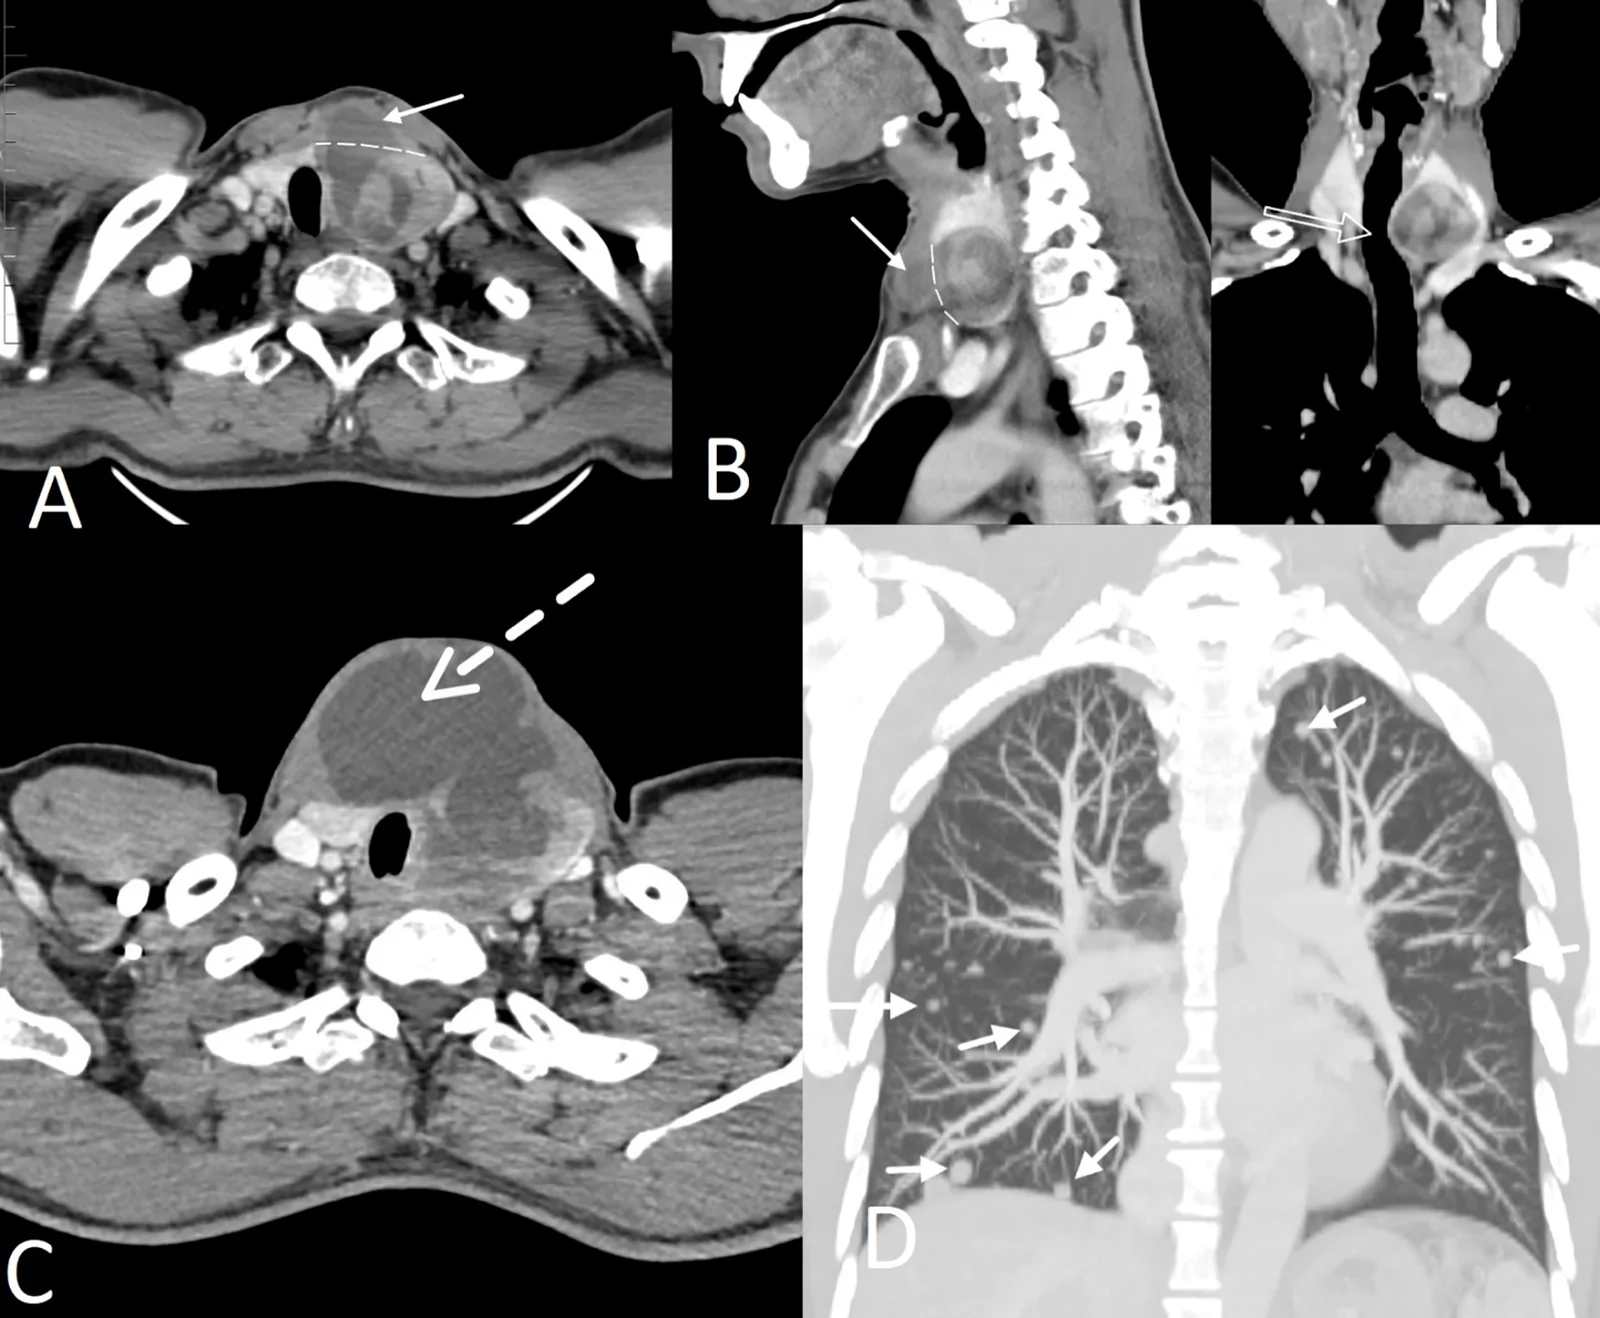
Cross-sectional imaging findings. (A, B) Contrast-enhanced CT of the neck in 2024 demonstrating a cystic left thyroid lesion with an enhancing solid component. The cystic component breached the anterior thyroid capsule with anteroinferior extrathyroidal extension posterior to the manubrium sterni. Mild rightward tracheal deviation and airway narrowing were present. (C) Follow-up contrast-enhanced CT in 2026 showing interval enlargement of the lesion, predominantly due to expansion of the inferior cystic component with retrosternal extension. The intrathyroidal and anteroinferior cystic components were contiguous and communicated with each other. (D) CT chest image demonstrating multiple bilateral pulmonary nodules consistent with metastatic disease. Suspected esophageal involvement on CT prompted contrast-enhanced MRI of the neck, which showed possible involvement of the left lateral esophageal wall.
